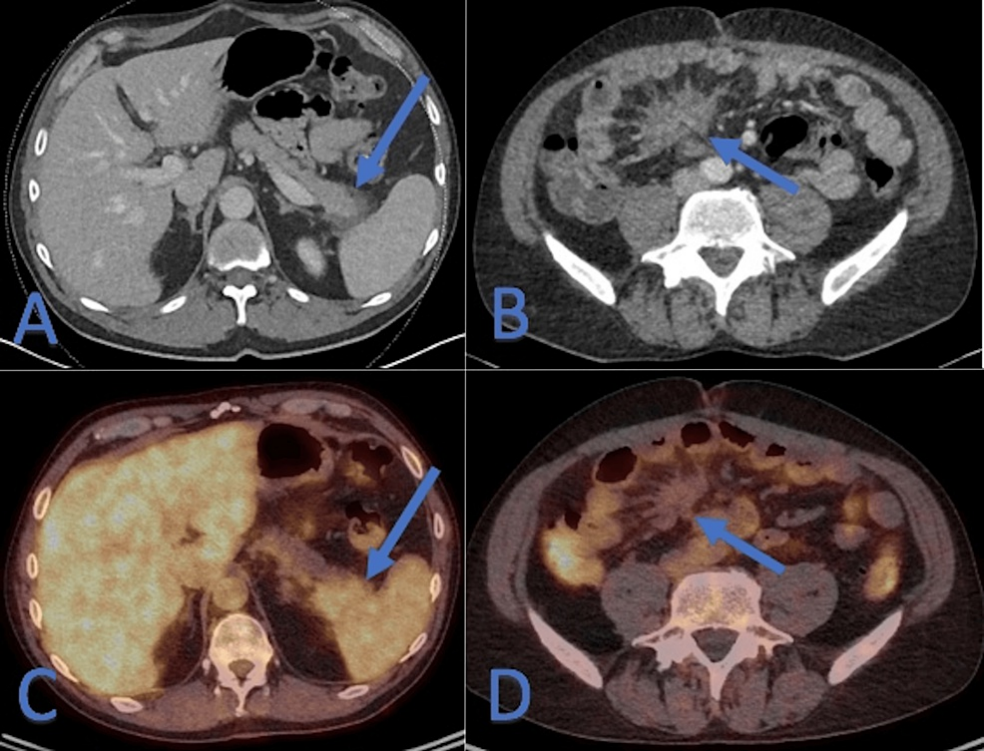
Six-week follow-up Contrast-enhanced computed tomography (CECT) (A and B) and F-fluorodeoxyglucose (FDG) positron emission tomography/computed tomography (PET-CT) (C and D) after six weeks of treatment. Size and activity decrease of the pancreatic and mesenteric masses (arrows in A, B, C and D); notice the recovery of the normal aspect of the pancreatic tail (arrow on A).
